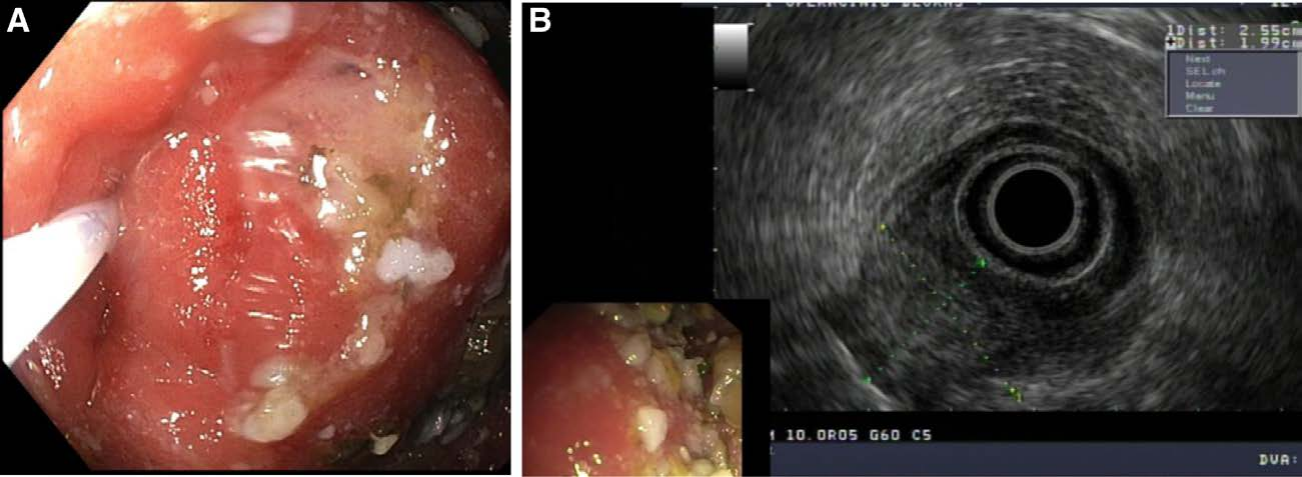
(A) Gastroscopy showing a submucosal lesion with intact mucosa causing pyloric stenosis. Note gastric contents in the lumen and a probe inserted in the stenotic pylorus. (B) Endoscopic ultrasound revealed an isoechoic submucosal lesion in the wall of gastric pylorus.
